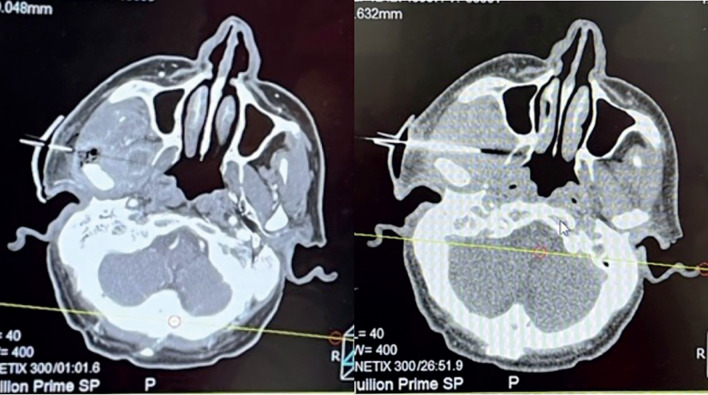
CT image during puncture needle insertion prior to collection drainage and CT image after collection drainage.
